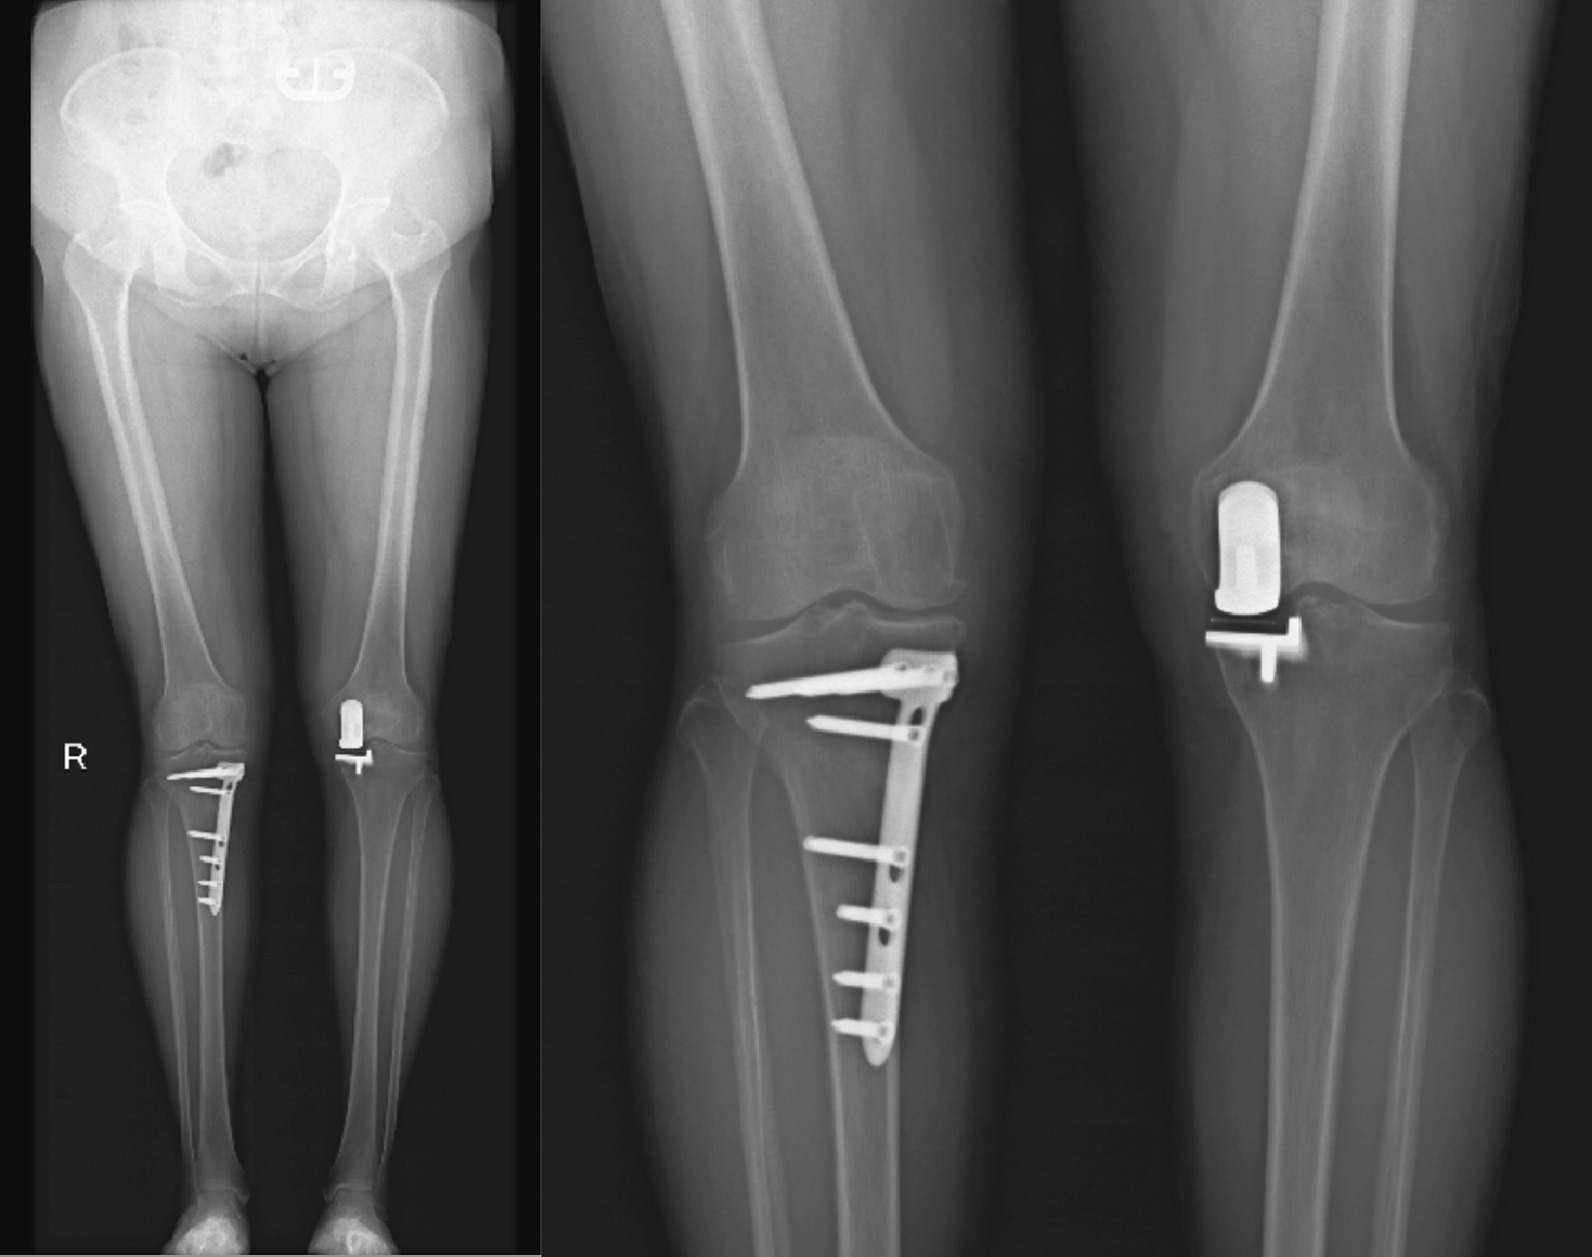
Postoperative images of HTO and UKA. A shows full-length radiographs of the lower limb, and B shows weight-bearing anteroposterior radiographs of the knee joint
